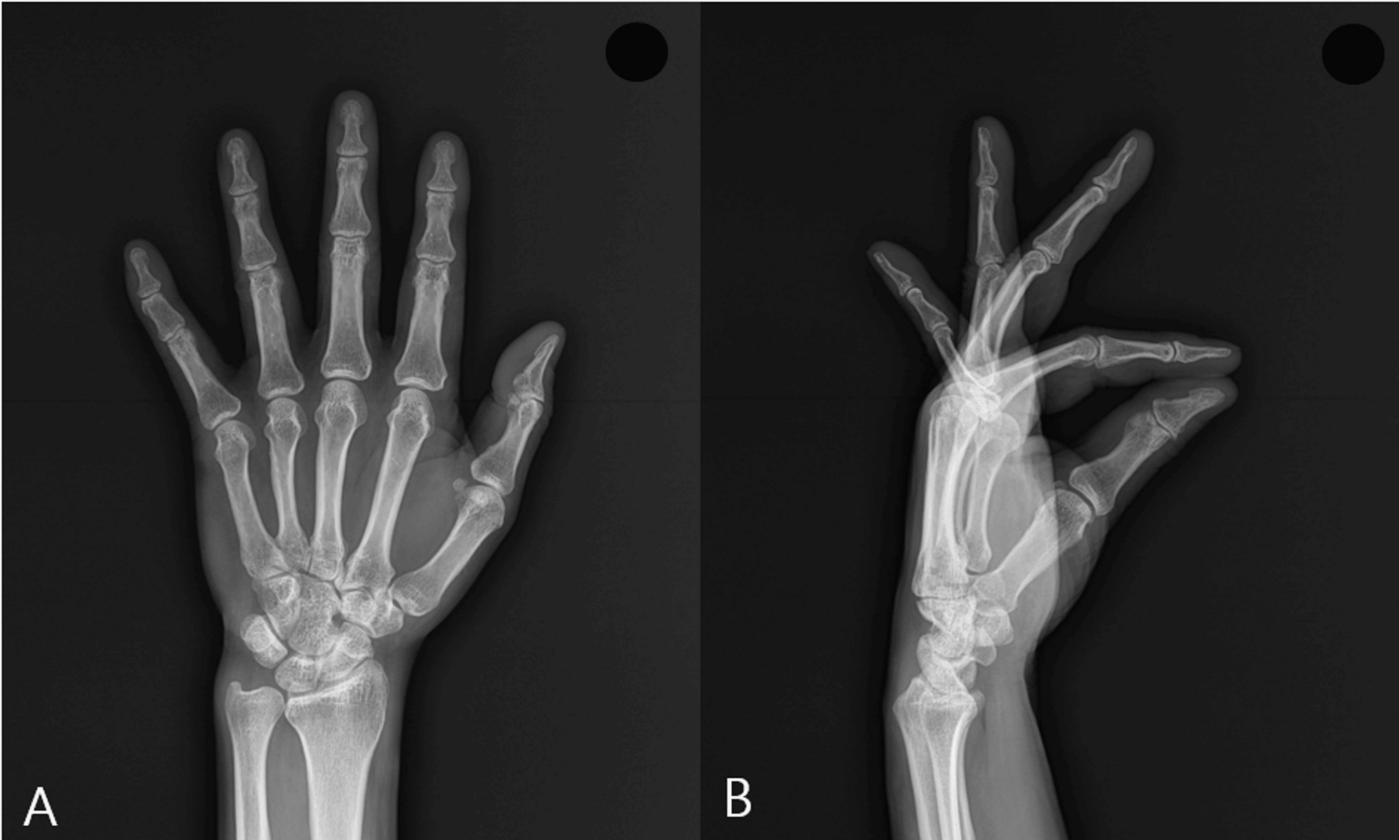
Posteroanterior (PA) (A) and lateral (B) radiographs of the wrist, obtained with the forearm in neutral rotation, demonstrate neutral ulnar variance on the PA view and no evidence of fracture, dislocation, or degenerative change on either view.
